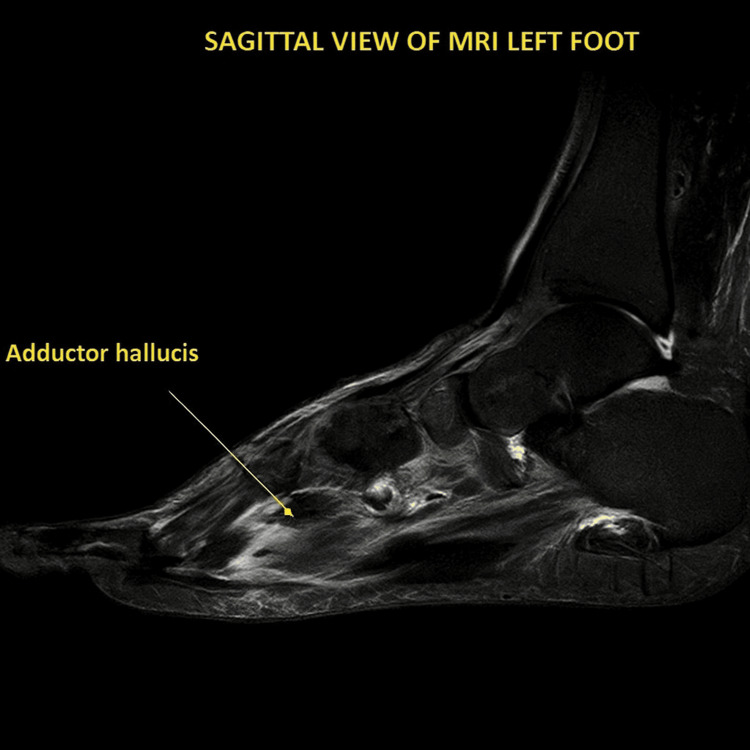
Sagittal STIR MRI of left foot STIR: short-TI inversion recovery.
